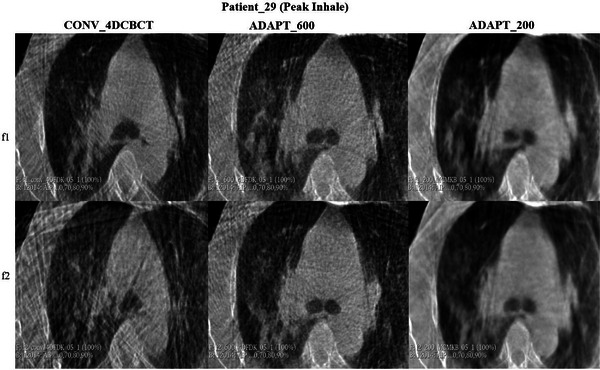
Visual assessment of tumor motion for patient_29 during peak inhale phase. The comparison includes conventional 4DCBCT, ADAPT_600, and ADAPT_200 scans across fractions 1 (f1) and 2 (f2).
